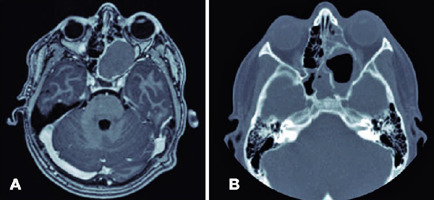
Preoperative magnetic resonance imaging (MRI) and postoperative computed
